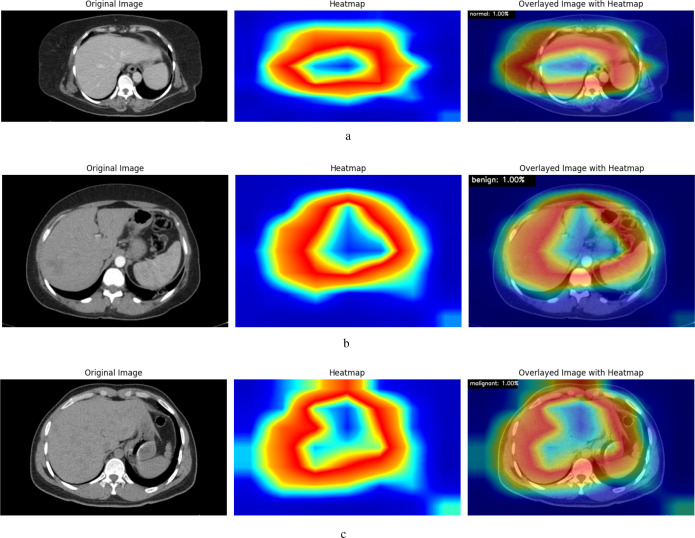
Grad-CAM visualizations of CT liver tumor predictions. Class activation maps for (a) Normal, (b) Benign, and (c) Malignant classes, highlighting discriminative image regions.
